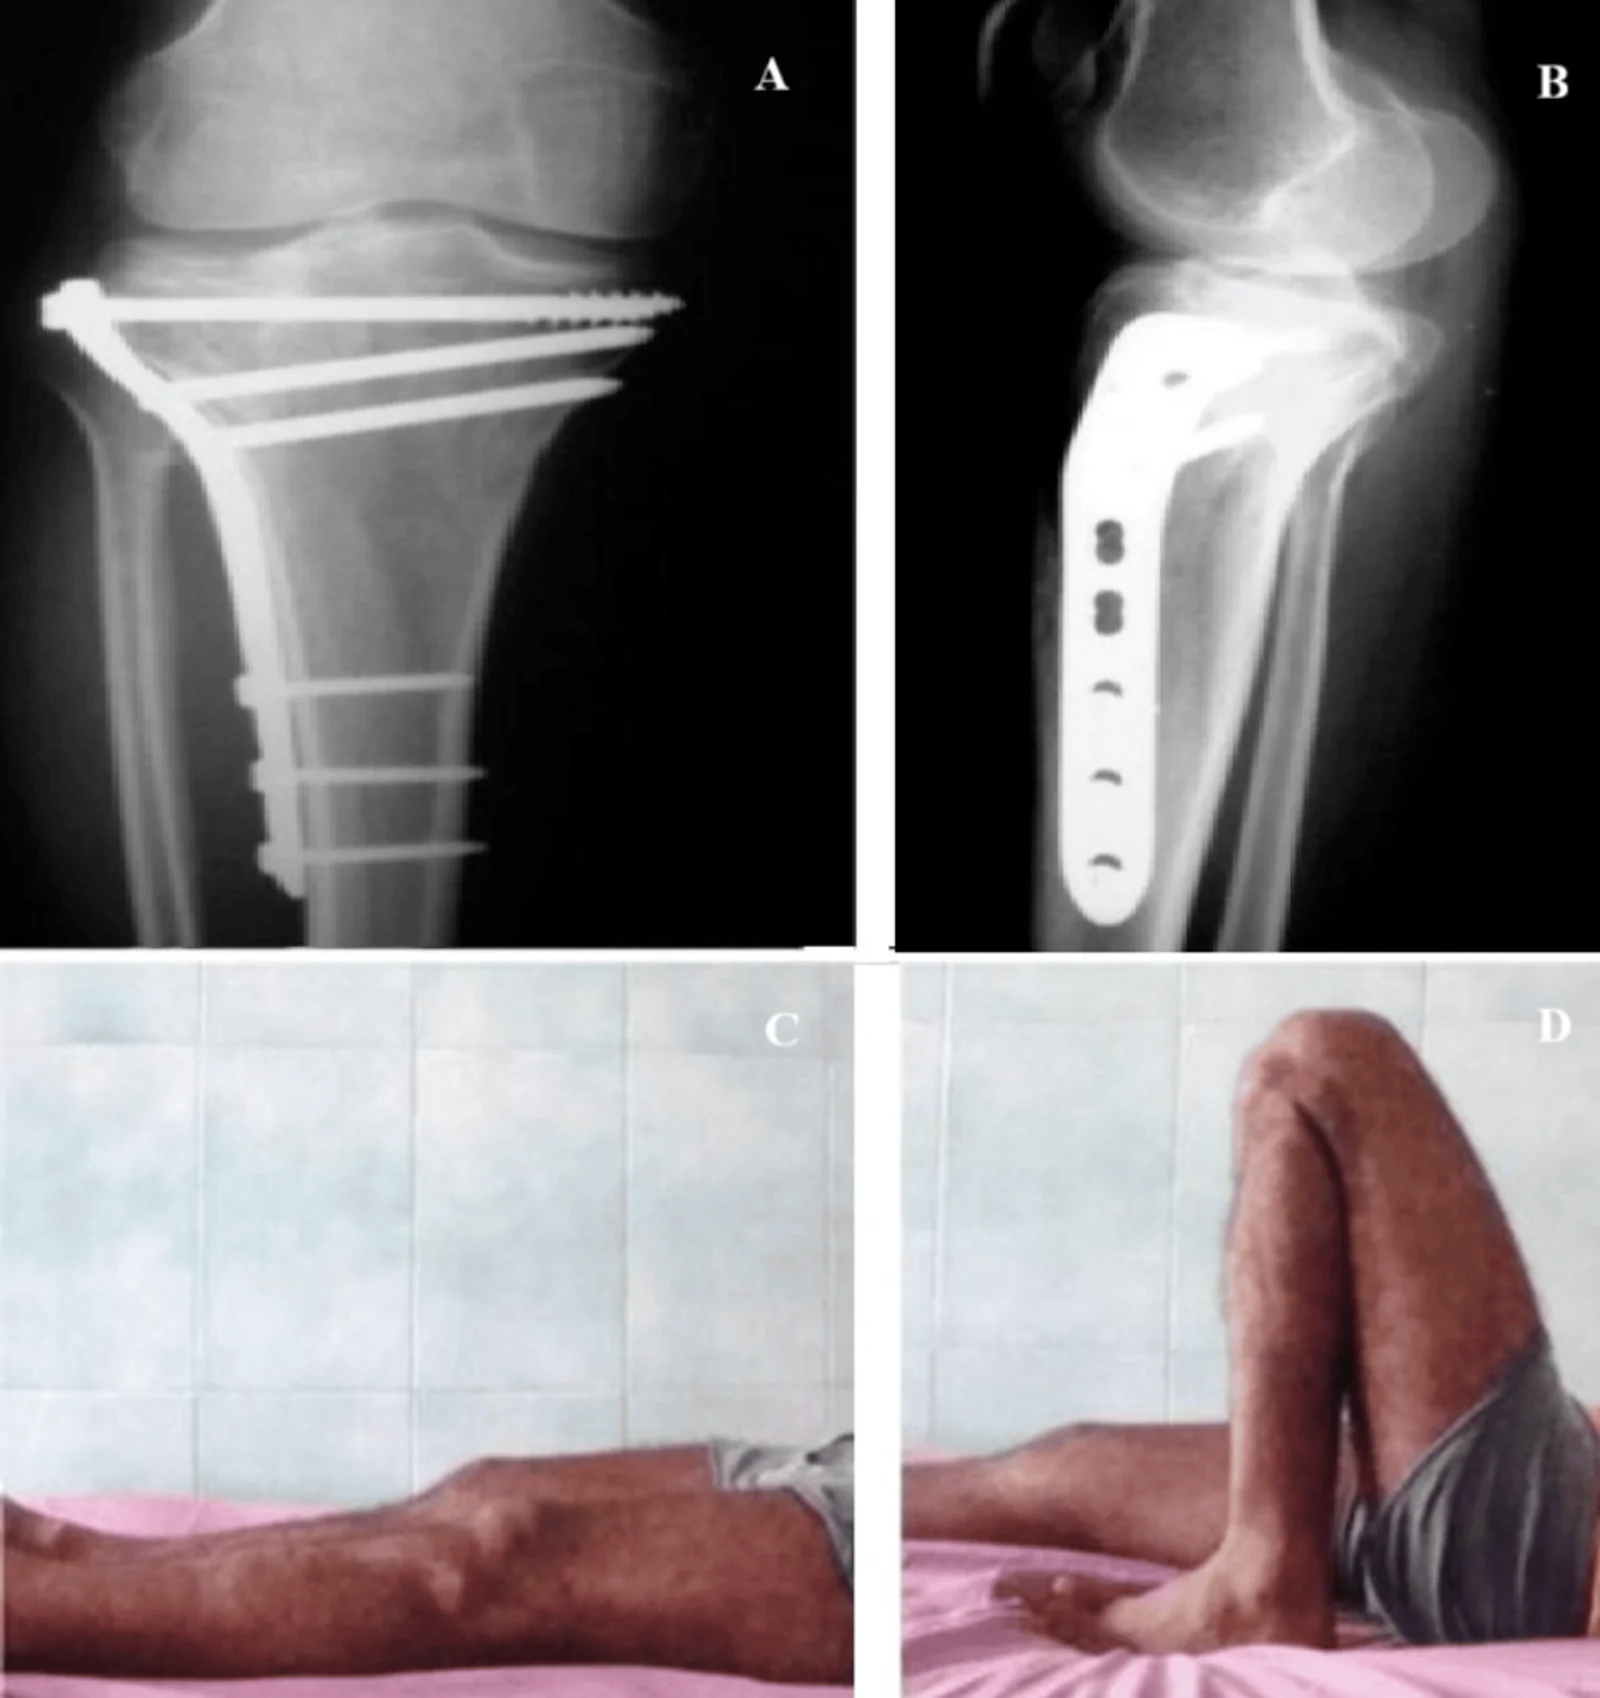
Bone grafting done for the depressed proximal tibia fracture Postoperative (A) anteroposterior and (B) lateral radiographs demonstrating bone grafting and fixation of a depressed proximal tibial fracture. (C,D) Clinical photographs demonstrating postoperative knee range of motion
